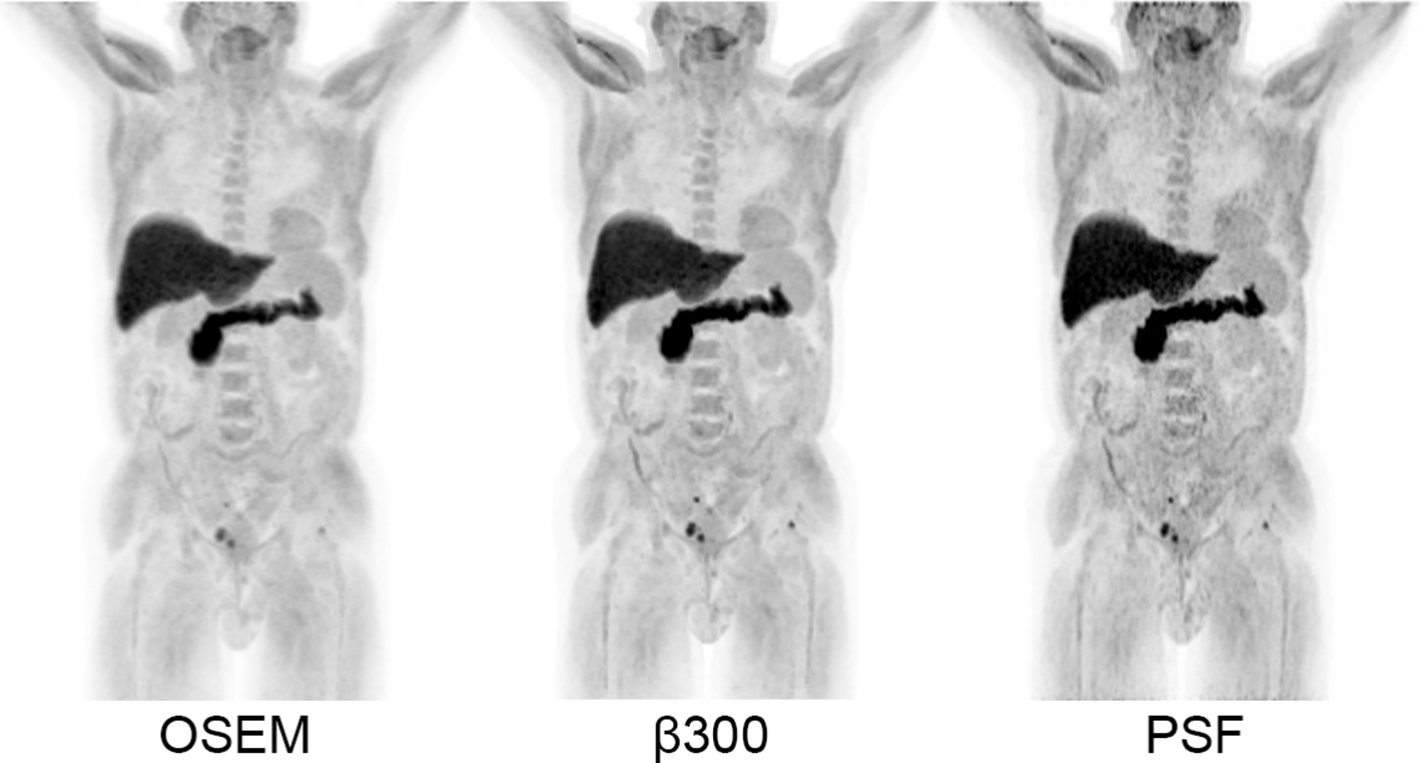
Maximum intensity projection images (SUV scale 0–7) of a patient demonstrating small foci of disease recurrence in the pelvis and left femoral head, rendered more conspicuous on the BPL β 300 reconstruction compared to OSEM, but without the penalty of increased noise depicted on the PSF reconstruction. OSEM, ordered subset expectation maximisation; PSF, point spread function; SUV, standardised uptake value.
